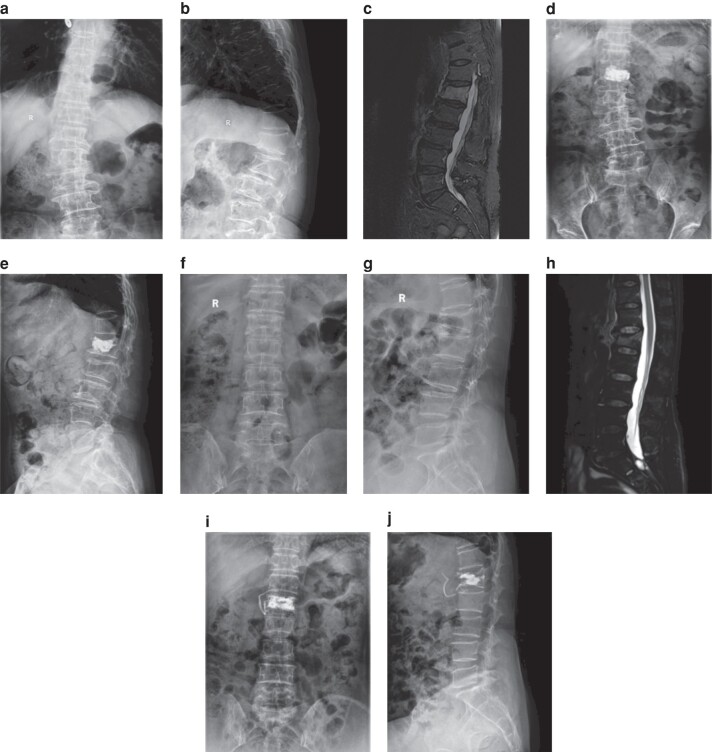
Percutaneous vertebroplasty (a-e) and percutaneous kyphoplasty (f-j) Percutaneous vertebroplasty: a AP thoracolumbar X-ray before surgery. b Lateral thoracolumbar X-ray before surgery. c Sagittal thoracolumbar MRI before surgery. d AP thoracolumbar X-ray post-PVP surgery. e Lateral thoracolumbar X-ray post-PVP surgery. AP, anterior-posterior; PVP, percutaneous vertebroplasty. Percutaneous kyphoplasty: f AP thoracolumbar X-ray before surgery. g Lateral thoracolumbar X-ray before surgery. h Sagittal thoracolumbar MRI before surgery. i AP thoracolumbar X-ray post-PKP surgery. j Lateral thoracolumbar X-ray post-PKP surgery. AP, anterior-posterior; PKP, percutaneous kyphoplasty; R, right.
